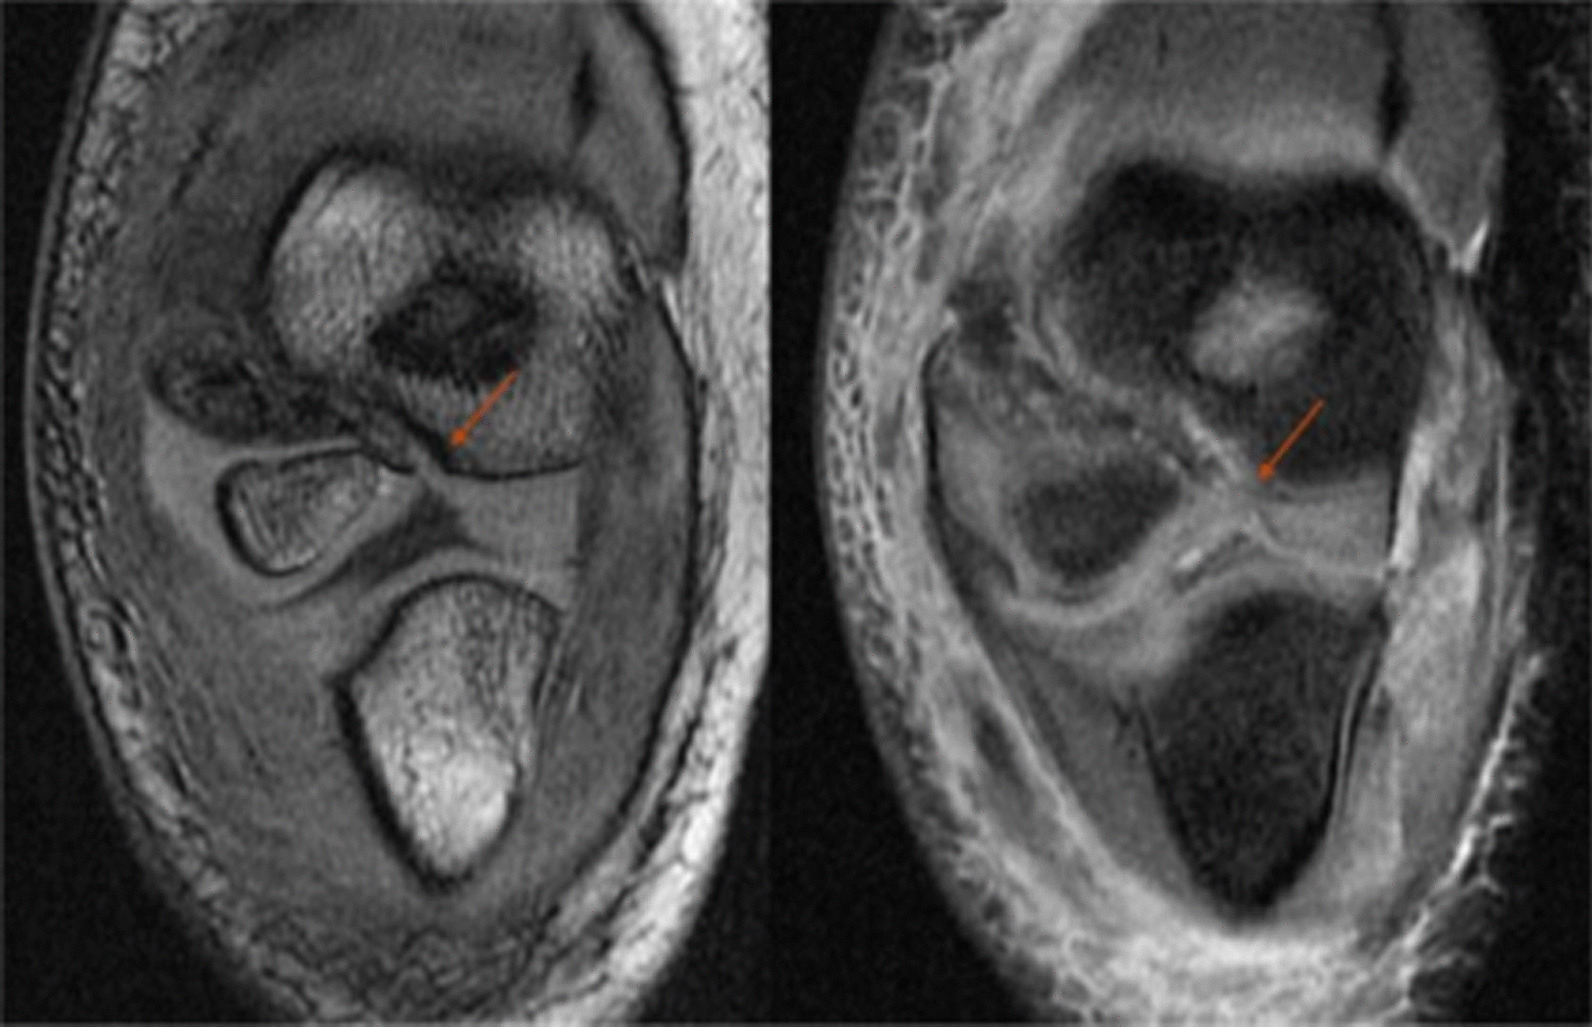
The MRI of right elbow in a 7 years old boy with HLCF. It showed slightly displaced fracture (< 2 mm) by radiograph. MRI showed that the fracture line involved the humeral trochlear cartilage chain, the cartilage articular surface was broken and the fractured end was displaced (3.7 mm) (Left: coronal 3D-FSPGR; Right: coronal T2 fat-suppression; The arrow showed the fracture line). HLCF, Humeral lateral condyle fracture
